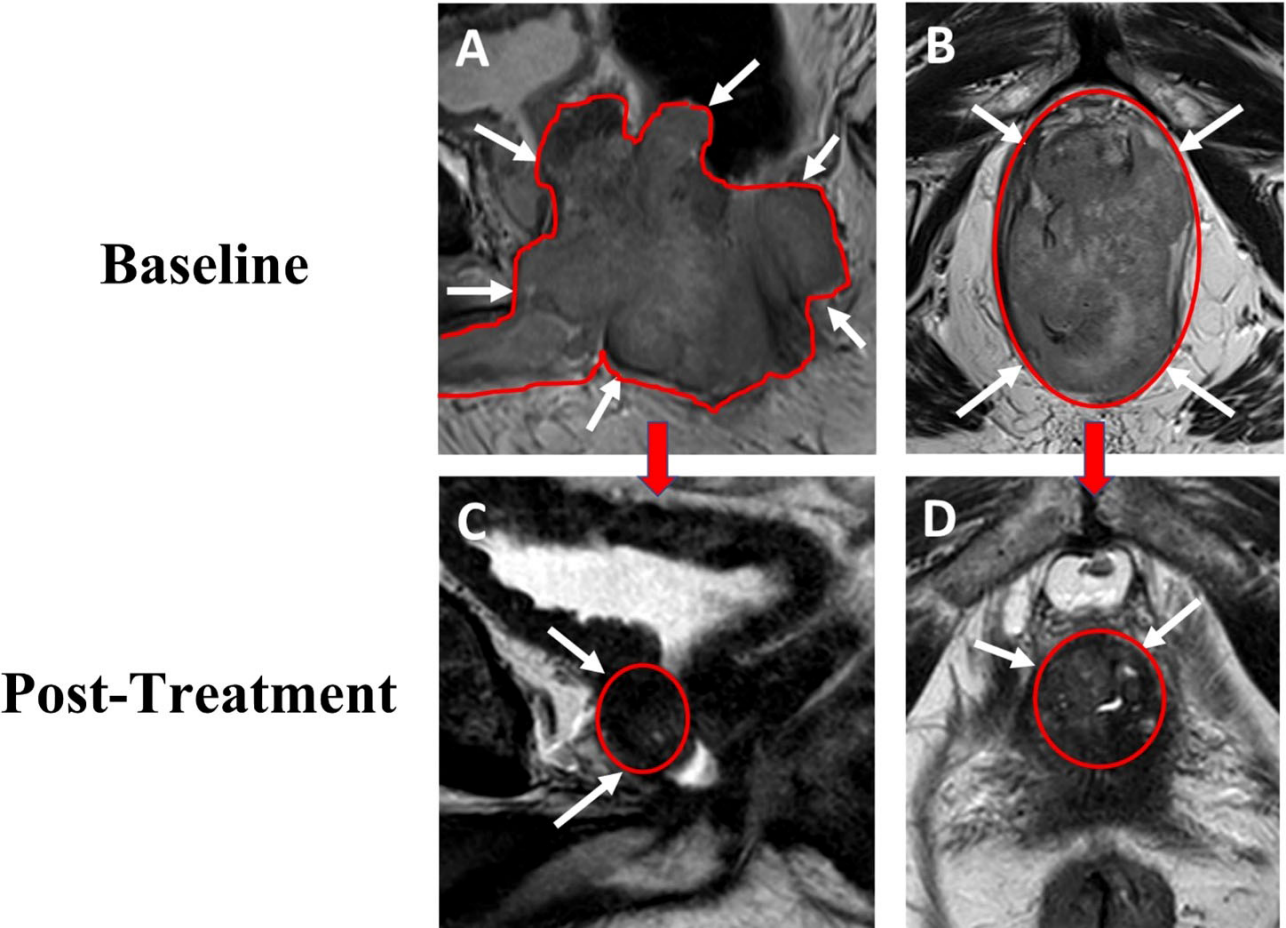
69-year-old male with advanced prostate cancer. Baseline sagittal (A) and axial (B) T2W MRI performed after transurethral resection show a large mass (total lesion volume of 282cc) occupying the whole prostate with rectum, bladder with diffusion restriction on ADC map. Post-treatment follow up sagittal (C) and axial (D) T2W MRI show a gradual decrease in size of the lesion (total lesion volume 11.4cc) without evidence of rectum and bladder involvement. T2W, T2 weighted image; ADC, apparent diffusion coefficient.
